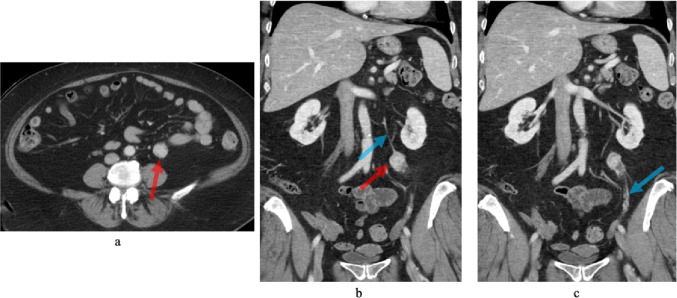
Leiomyosarcoma of the left gonadal vein. Axial (a) and coronal (b, c) contrast-enhanced CT images show an ovoid heterogeneously enhancing left retroperitoneal mass (red arrows) along the course of the left ovarian vein (blue arrows)
